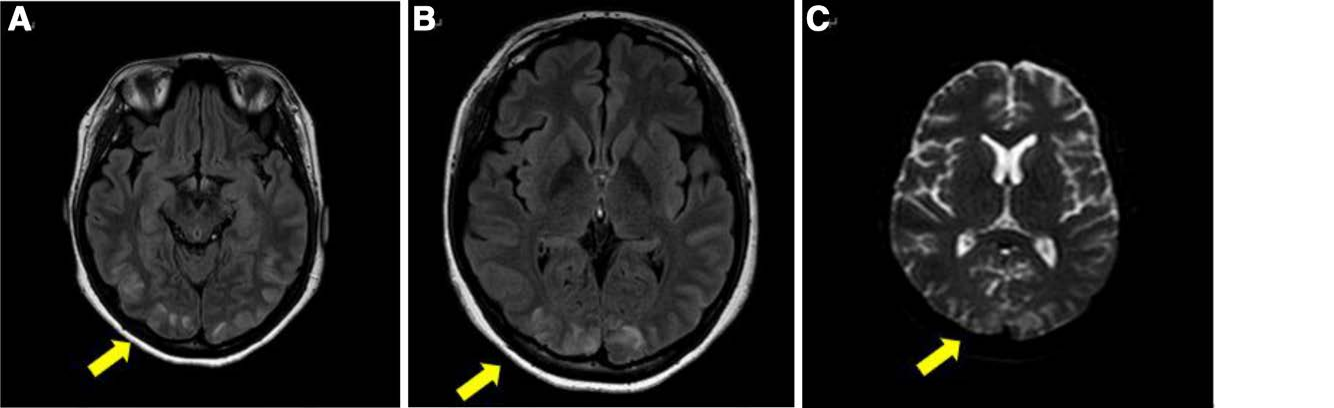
MRI performed on the day of visit. T2/FLAIR image showed high signal intensity in the parietal-temporal (A) and occipital regions (B) on both sides. High-intensity signal findings are seen on DWI image (C). DWI = diffusion-weighted imaging, MRI = magnetic resonance imaging.
